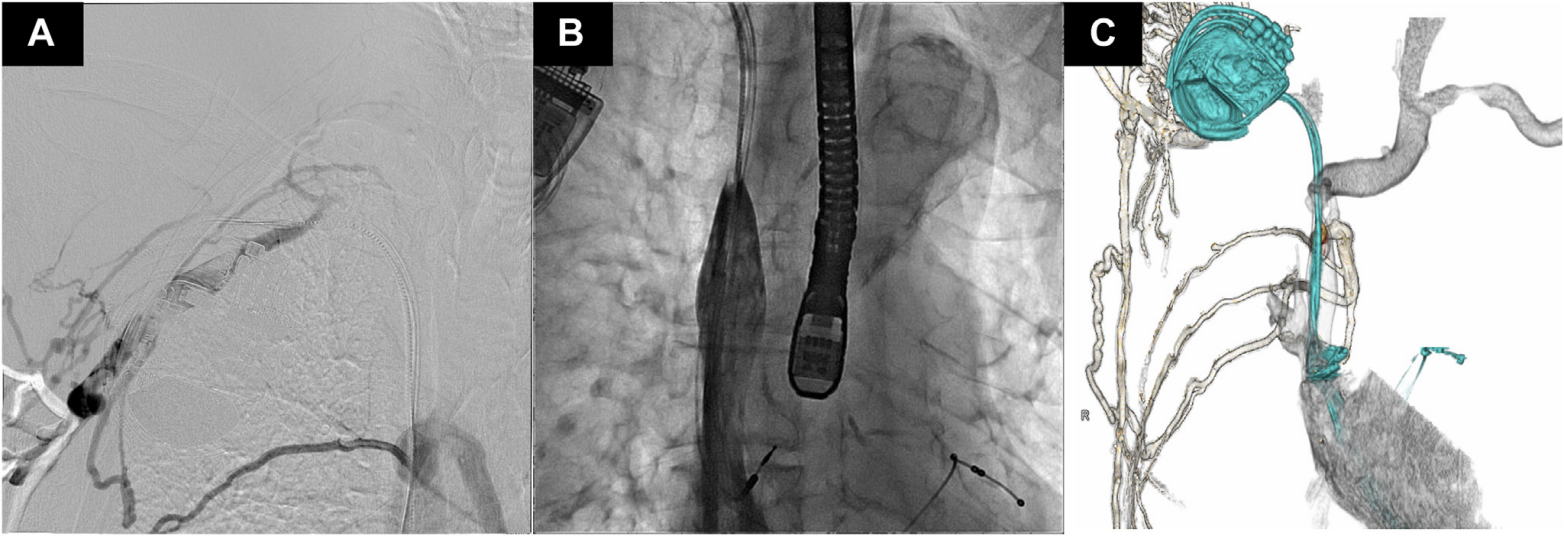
Preprocedural images. A: Digital subtraction venography showing the occluded right brachiocephalic vein and upper portion of the superior vena cava. B: Retrograde venography showing the occluded upper superior vena cava. C: Computed tomography revealing communication between the right axillary and the azygos veins via the intercostal veins. The azygos vein became the main collateral, draining into the superior vena cava.
